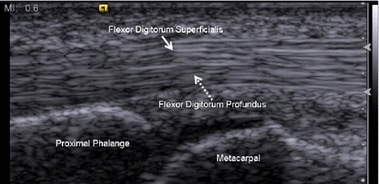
Finger ultrasound image at the A1 pulley
